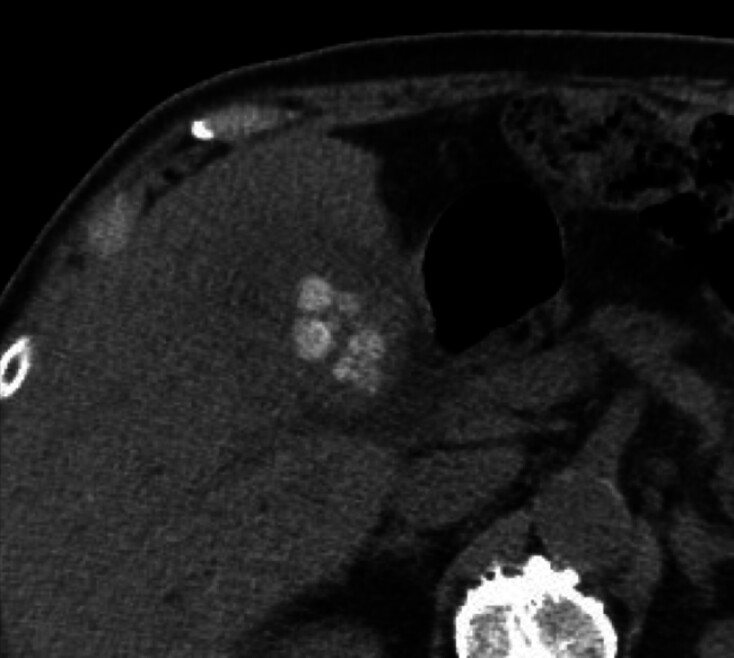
Computed tomography showed gallstones and common bile duct stones.
